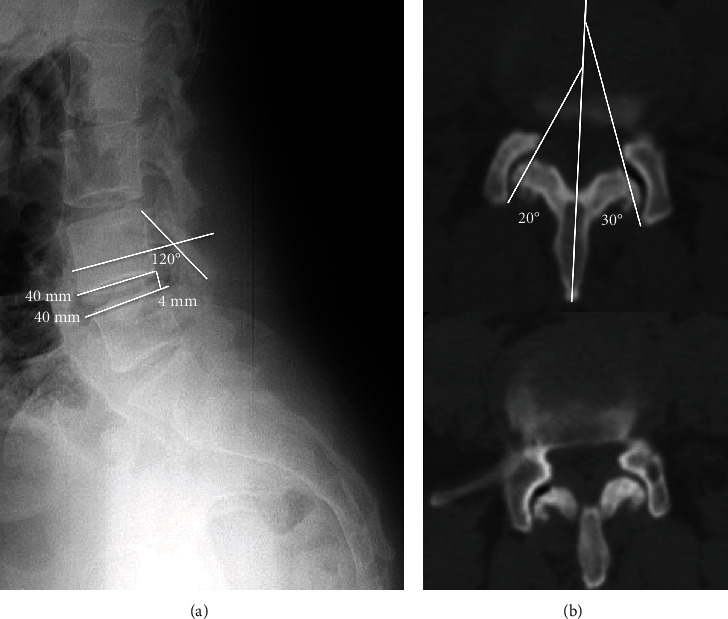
(a) Standing lateral radiograph where L4-L5 segmentary instability (4 mm) can be seen. (b) Laminar inclination angle. In (a), we can appreciate vacuum phenomenon and facet hypertrophy, with 10° of facet tropism that suggests segmentary instability.
